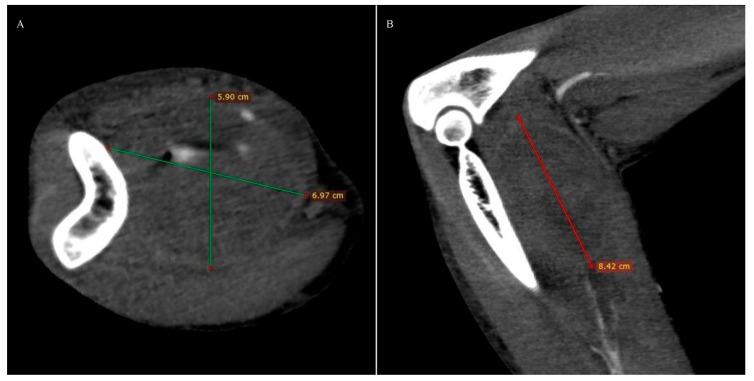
Right upper limb computed tomography angiography. MPR reconstruction. Soft tissue hematoma around the brachial artery: (A). Axial view. (B). Coronal view.
